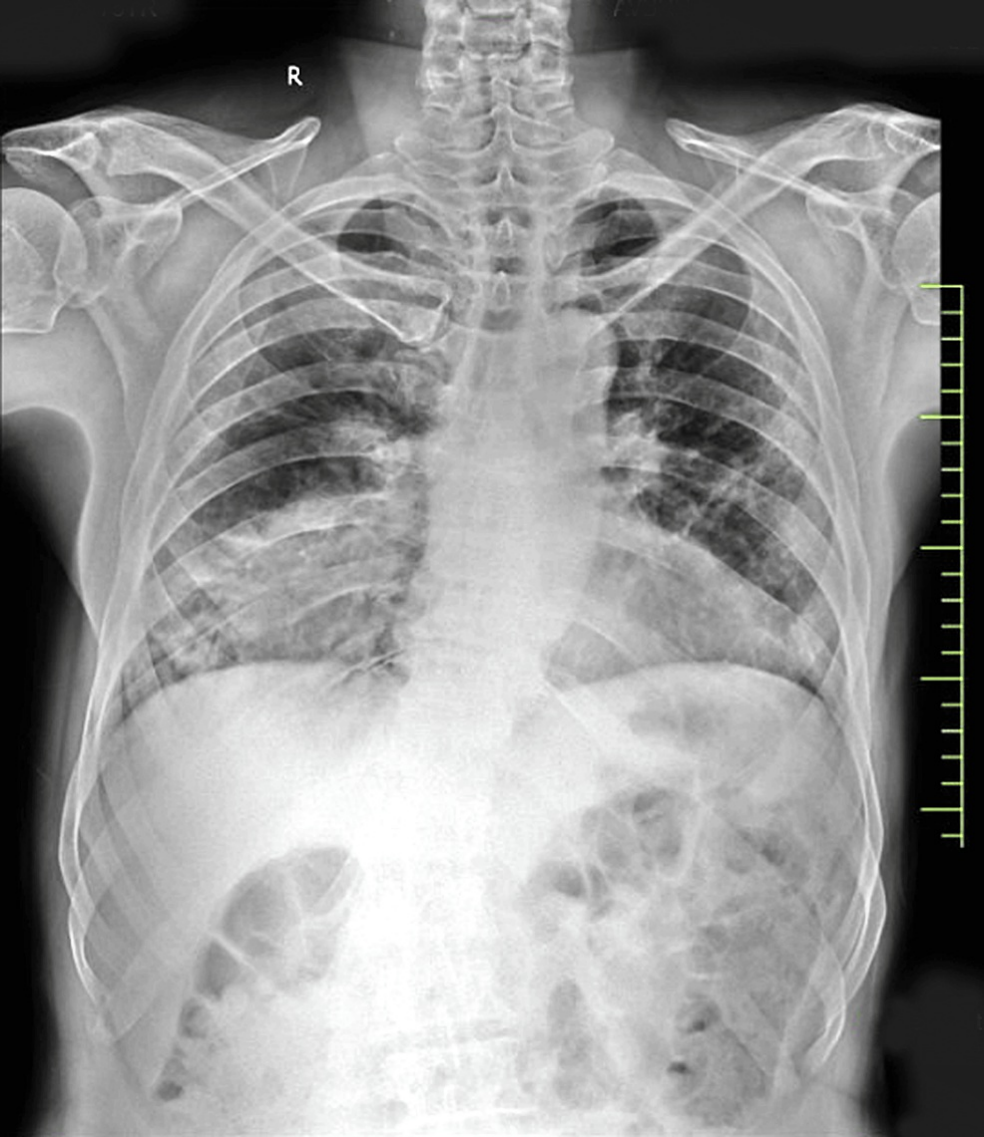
Chest radiograph of the patient on presentation. Homogenous rounded consolidation in the right lower zone of the lung.
